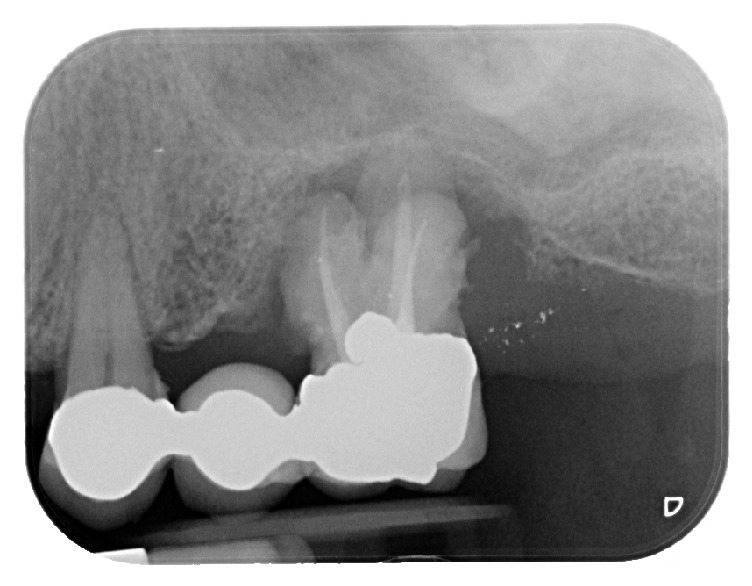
Intraoral radiograph at the patient presentation. Tooth 26 is affected by an endoperiodontal lesion and is lost.
